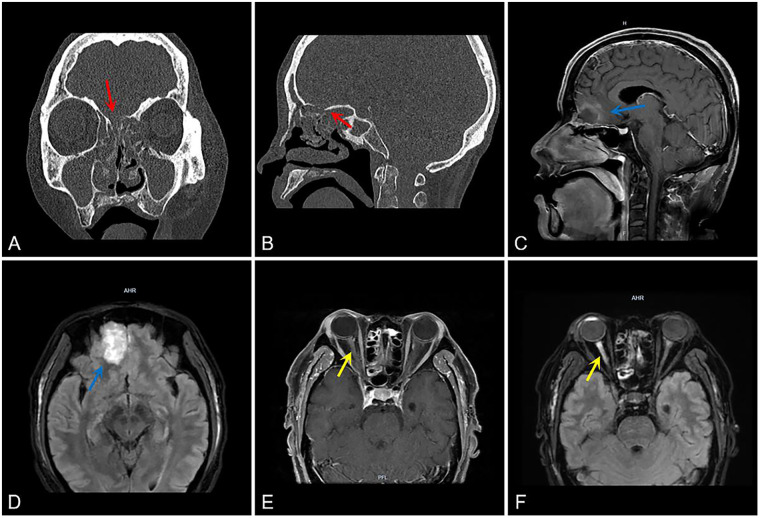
Imaging findings. Sinus CT shows sinus mucosal thickening with associated soft tissue density, along with bony destruction of the left orbital wall and skull base (A,B) (red arrows). Cranial MRI reveals intracranial abscess formation (C,D) (blue arrows). Cranial MRI demonstrates irregular soft tissue opacity in the right ethmoid sinus and optic nerve edema (T2-Flair hyperintensity) with mild enhancement (E,F) (yellow arrows).
